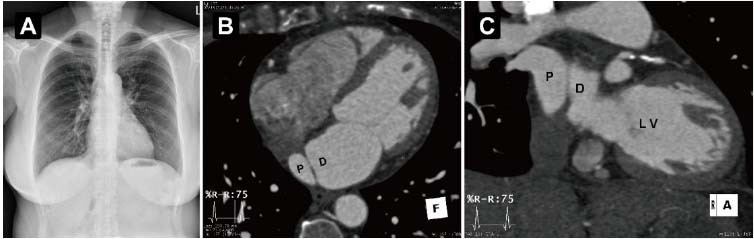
Comparison imaging from a patient with Cor Triatriatum Sinister (CTS) but without chronic adhesive pericarditis. (A) Chest radiograph showing no evident pericardial calcification. (B, C) Cardiac computed tomography angiography revealing an accessory septum dividing the left atrium into proximal (P) and distal (D) chambers. LV indicates the left ventricle.
